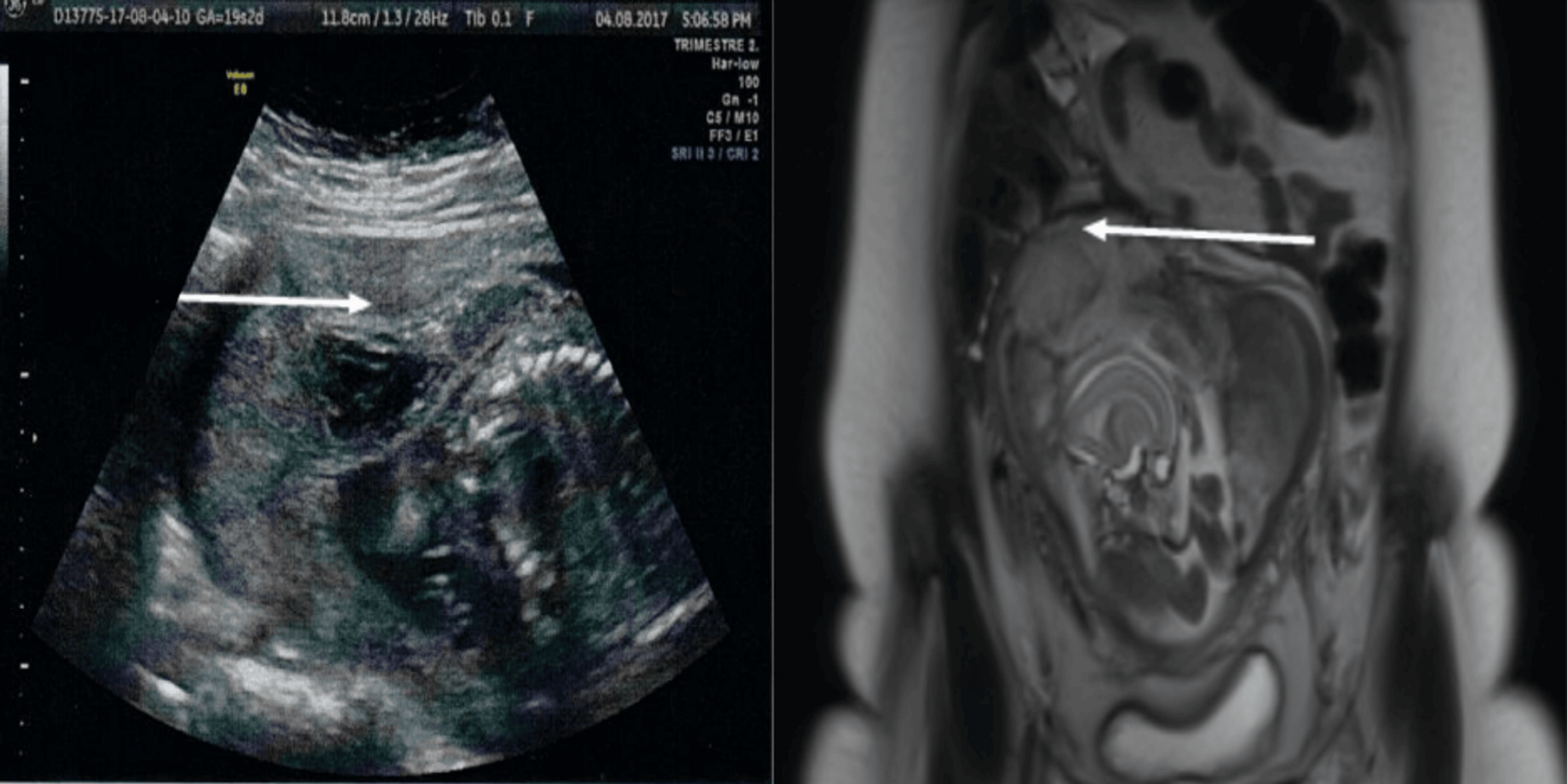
(Left) Central placental hematoma at 19 weeks of gestation and appearance of placenta accreta; (right) MRI sagittal view showing signs of fundal placenta accreta. MRI, magnetic resonance imaging
